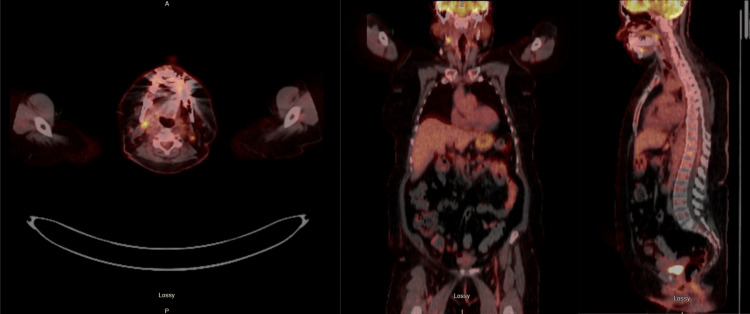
Case 1 FDG-PET CT of the skull base to the thigh Case 1 FDG-PET CT of the skull base to the thigh showed indeterminate FDG-avid left greater than the right cervical chain lymph nodes FDG: fluorodeoxyglucose
